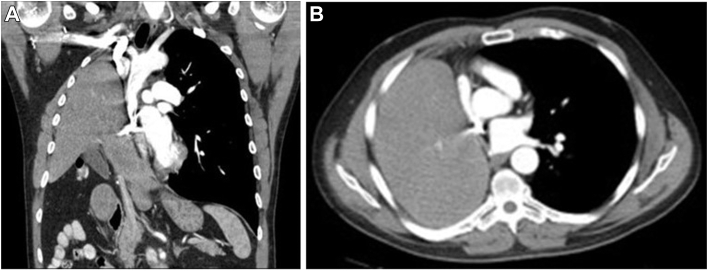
Preoperative chest computed tomographic scans. (A) Coronal image shows elevated right diaphragm with complete occupation of the pleural space with the liver and intrabdominal contents. (B) Axial image shows the intrathoracic liver exerting significant compression on the right atrium.
